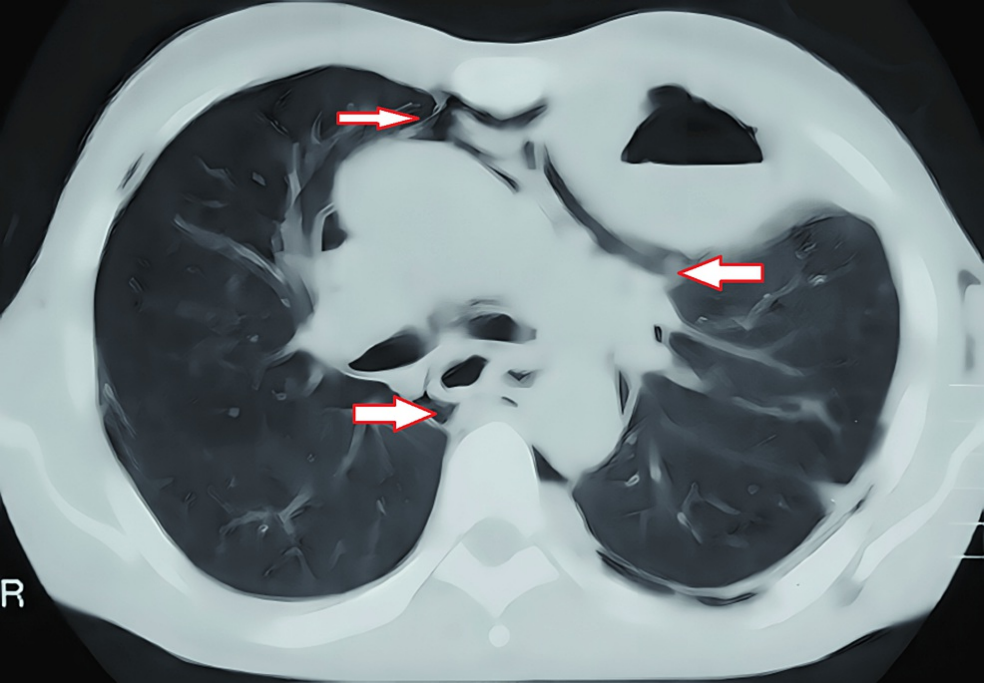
Computed tomography of the chest showing mediastinal emphysema with left loculated hydropneumothorax and subcutaneous emphysema around the trachea and neck.
